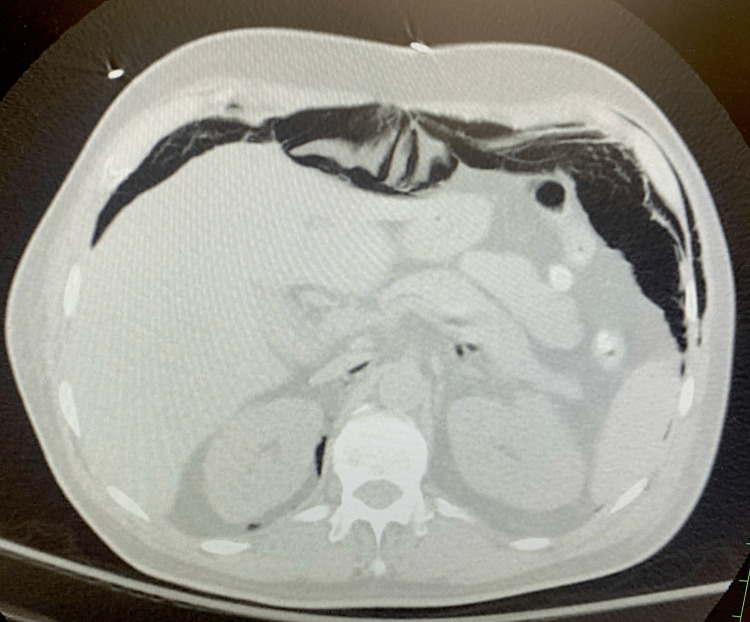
CT scan showing pneumoperitoneum in the patient.
